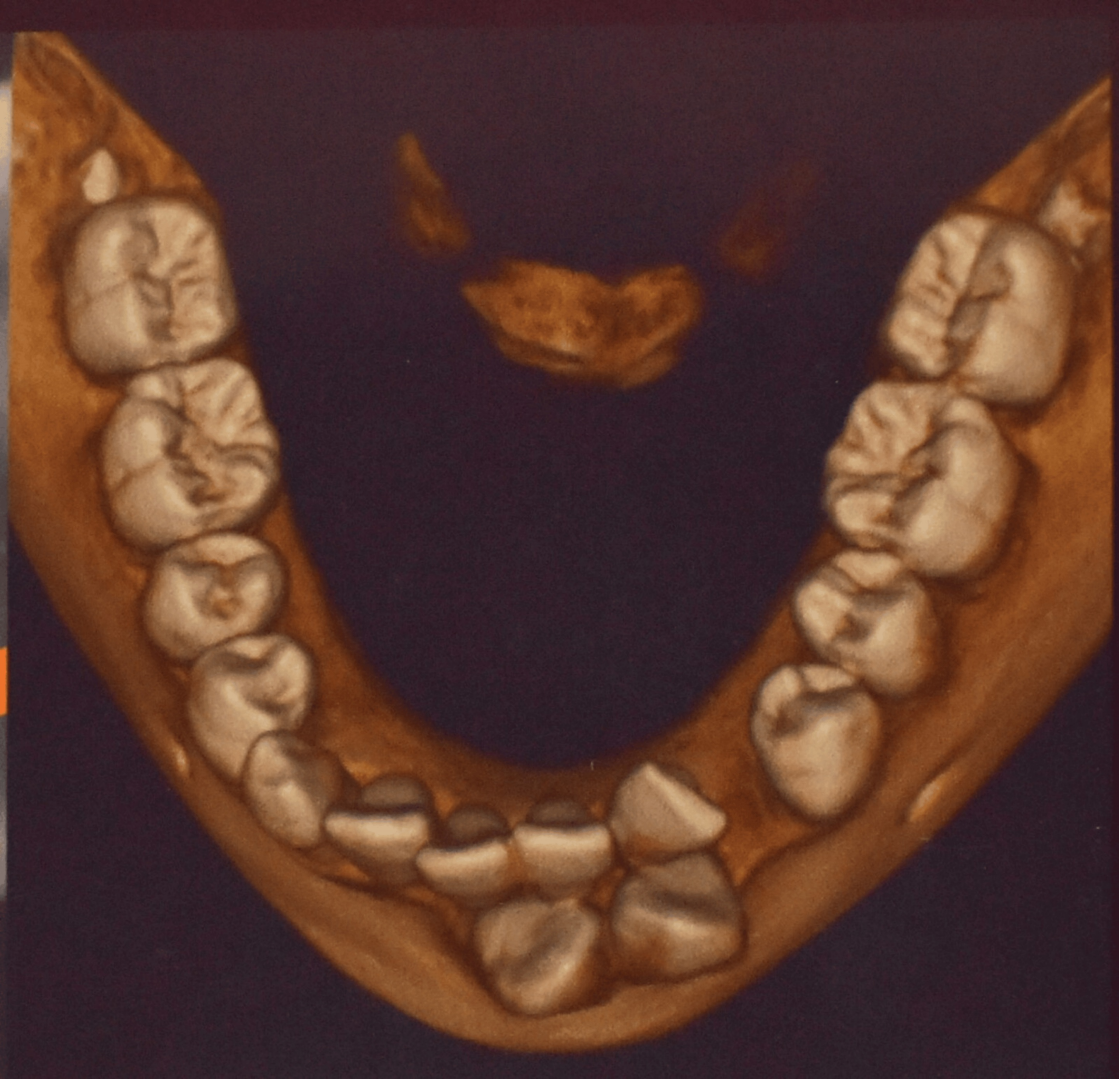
Occlusal view CBCT imaging of the mandible CBCT: cone beam computed tomography
